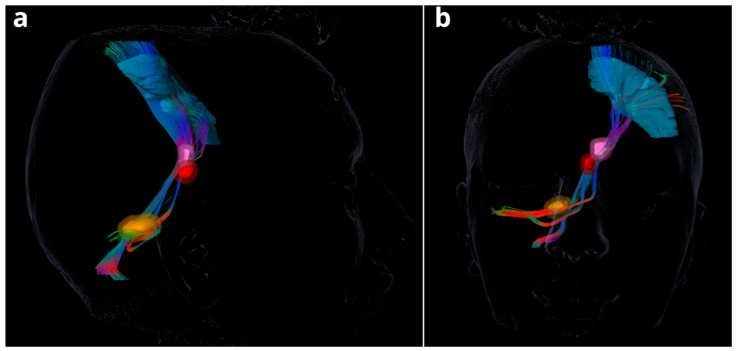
MRI Tractography of the Dentato-Rubro-Thalamic Tract (DRTt): (a) lateral view; (b) anterior–posterior view. DRTt starts in the dentate nucleus then courses through the superior cerebellar peduncle, and projects to the contralateral red nucleus and ventrolateral thalamic nuclei. Additionally, from the thalamus, fibers connect further to the primary motor cortex and premotor areas. Legend: Red—fibers running along the left–right (x) axis; Green—fibers running along the anterior–posterior (y) axis; Blue—fibers running along the superior–inferior (z) axis; while mixed colors indicate fibers with components in more than one direction, reflecting oblique trajectories.
